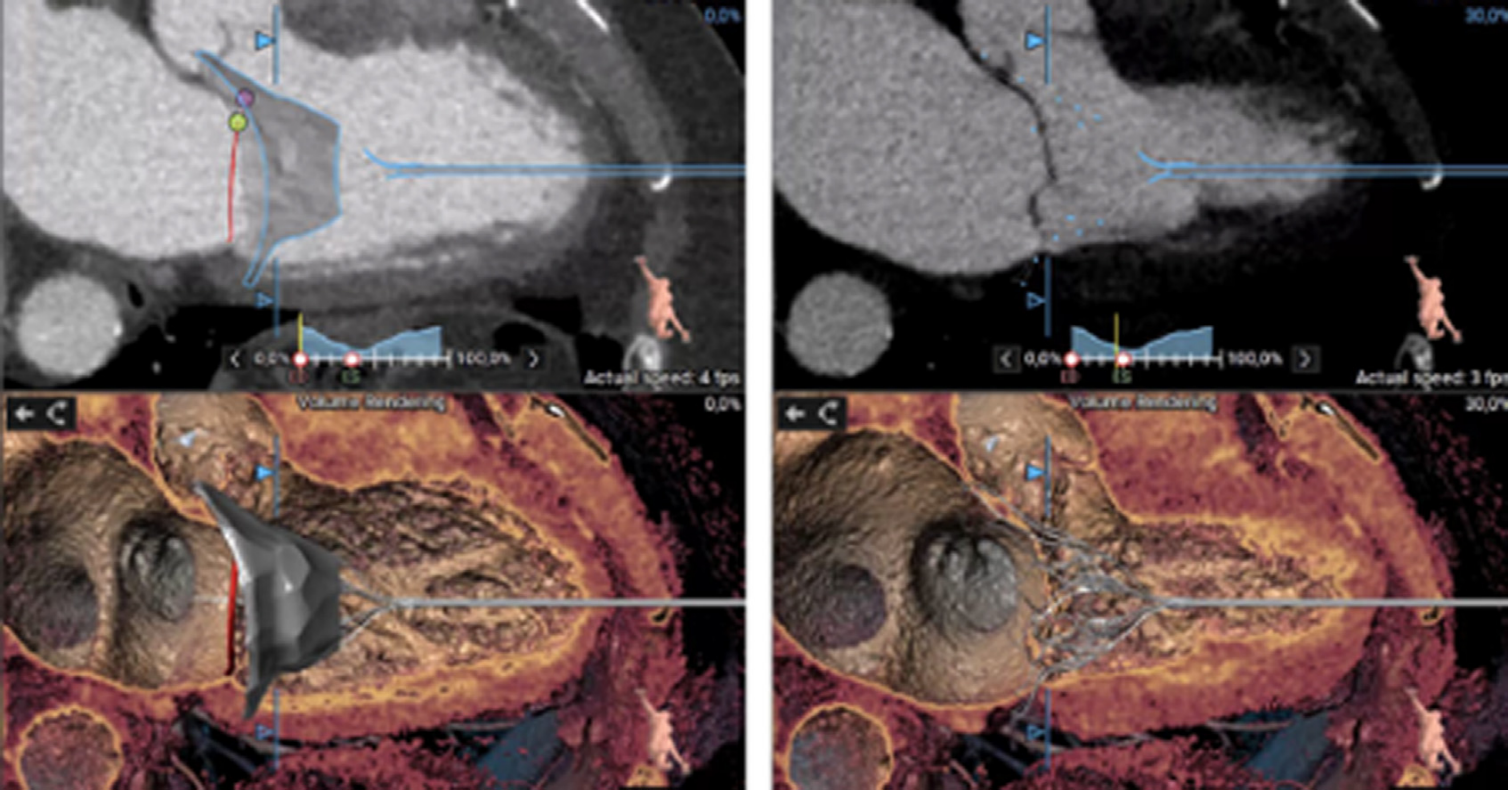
Cine Cardiac Computed Tomography Three-dimensional reconstruction of the position of the Valve 37M LP during systole and diastole.
